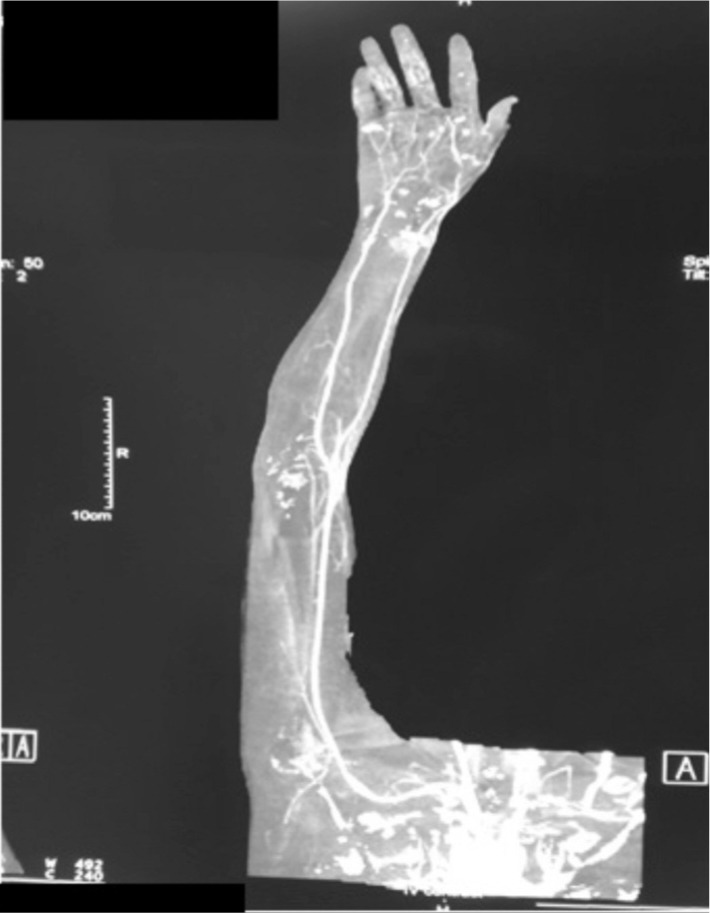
CTA right upper limb (at 6 weeks after jellyfish sting)
